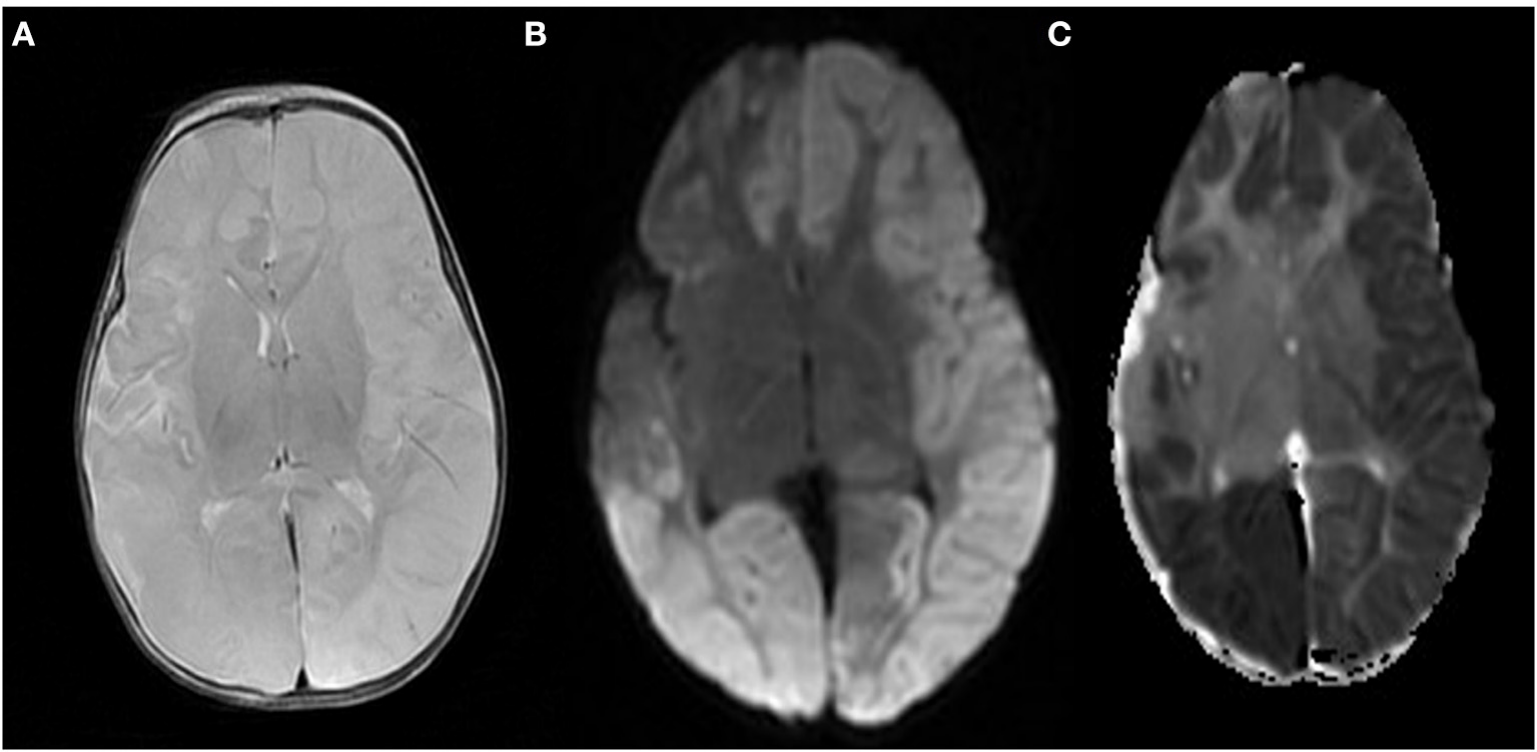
(A) MRI brain T2 weighted image that highlight cerebral oedema and cortical sulcal effacement. (B,C) Diffusion weighted image of the brain showing extensive areas of increased signal intensity with corresponding low ADC value, suggestive of hypoxic/ischemic brain injury.
